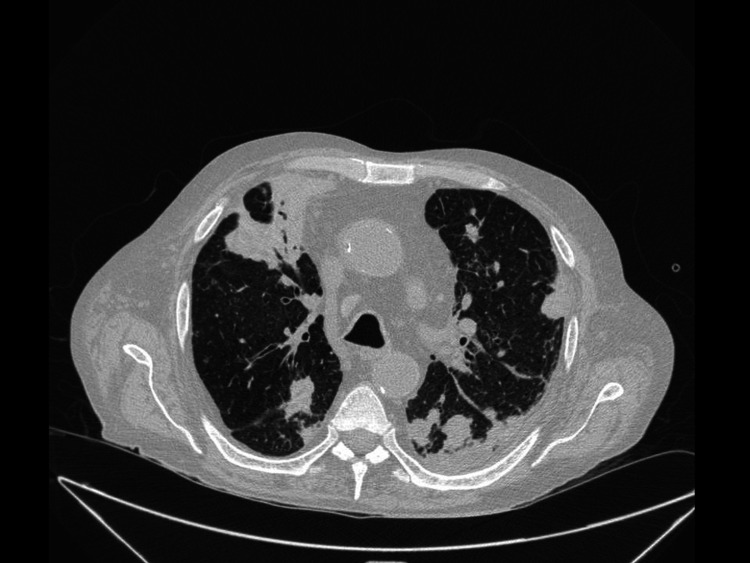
Chest CT scan showing pulmonary nodules and a left-sided pleural effusion
